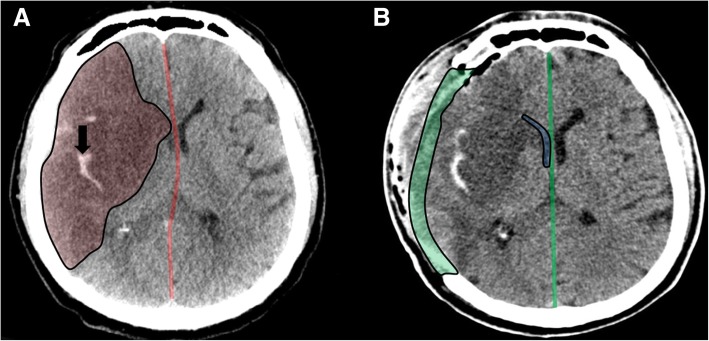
Decompressive hemicraniectomy for malignant ischemic stroke. Axial CT scan before surgery (a), demonstrating a demarcated right-sided MCA infarct (highlighted in red) with hemorrhagic transformation (black arrow) and midline shift to the left side (red line). Axial CT scan after surgery (b), showing the craniectomy defect (highlighted in green) with decompressed lateral ventricle (highlighted in blue) and reversal of midline shift (green line)
